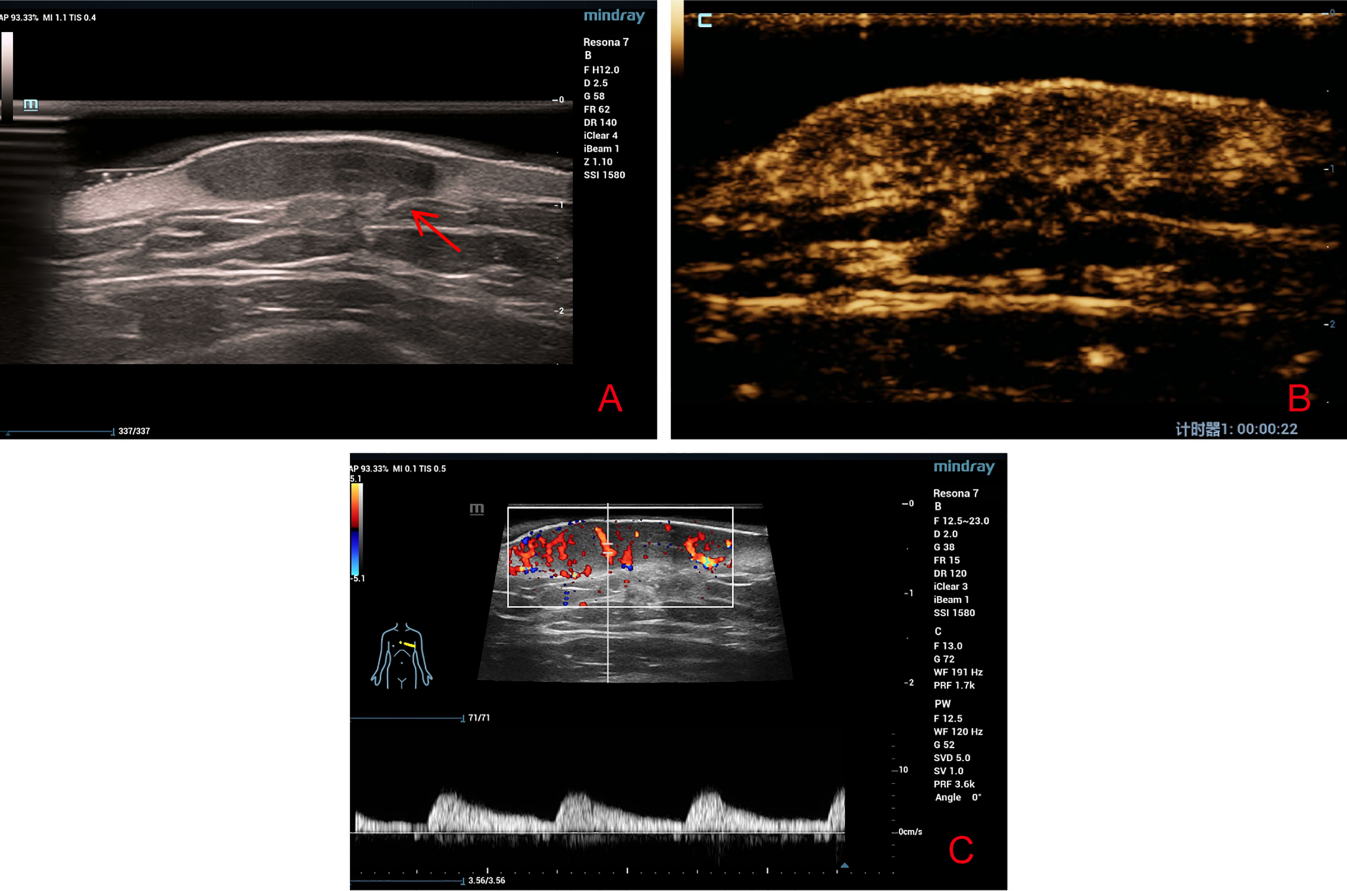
(A) High-frequency ultrasound revealed a well-defined irregular hypoechoic solid tumor in the dermis with protruding structures at some area that looked like a beak of a bird (red arrow). (B) Contrast-enhanced ultrasound revealed a heterogeneous high-enhancement pattern. (C) Color Doppler flow imaging revealed abundant blood flow signals around and inside the tumor.
